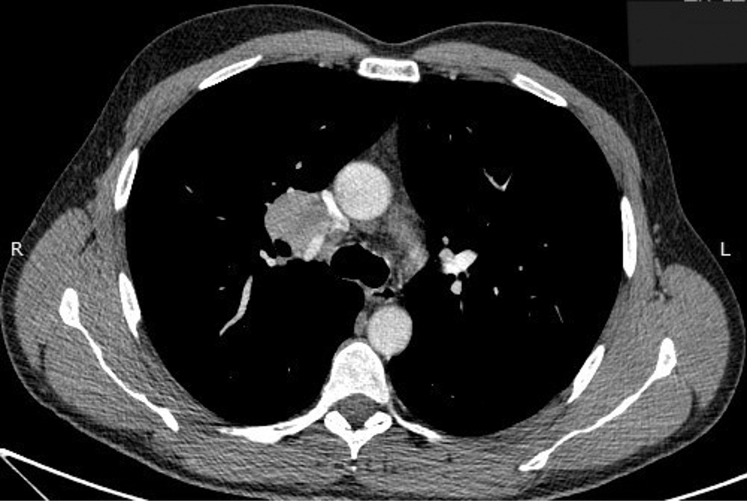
PET-CT scan showing a mildly hypermetabolic lesion at the right pulmonary hilum (standardized uptake value = 4.1).
